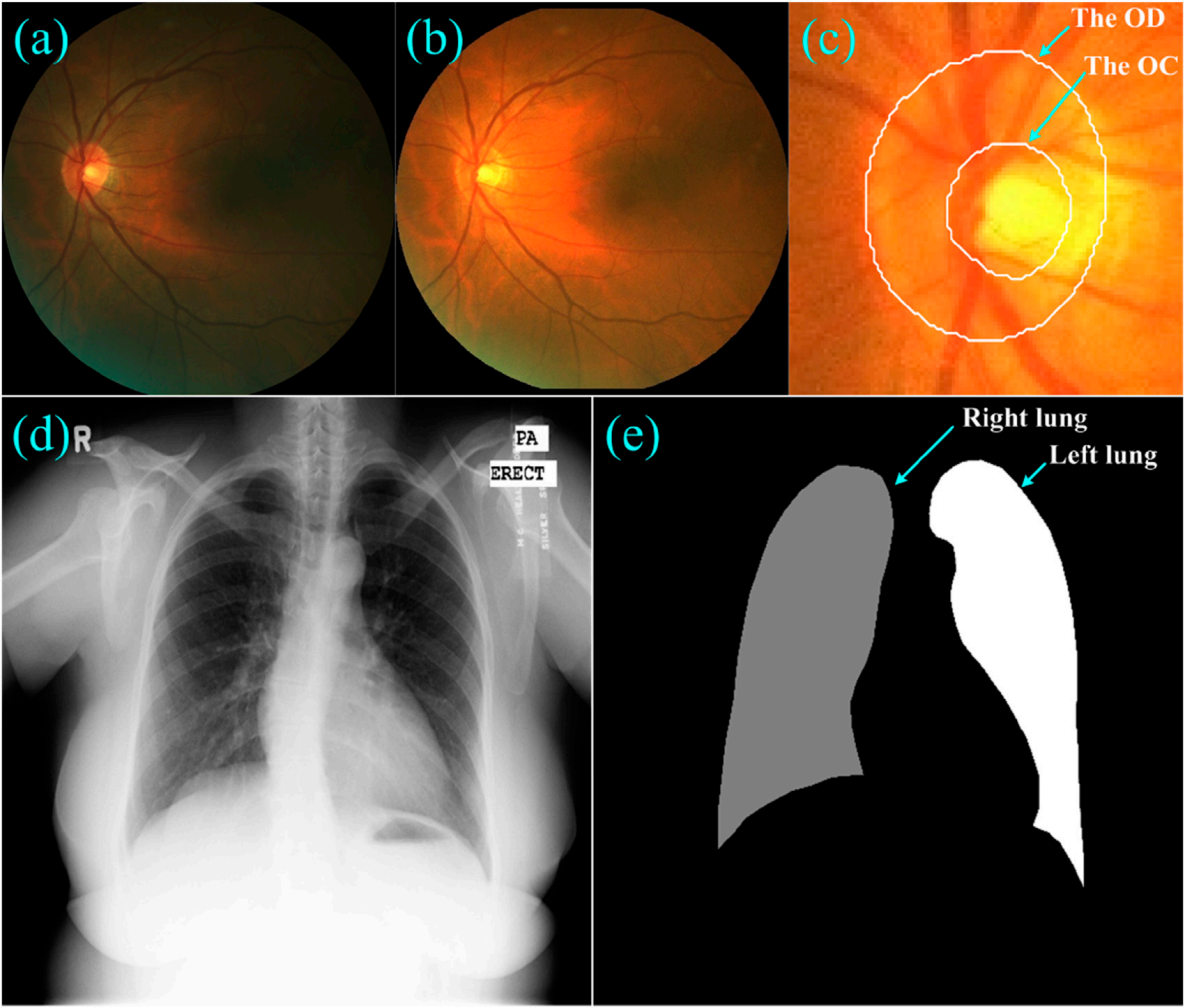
(A–C) showed a fundus image, its normalized version, and the local disc patch with manual annotations of the OD and OC, respectively, (D) and (E) showed a Xray image and its annotations for the left and right lungs.
